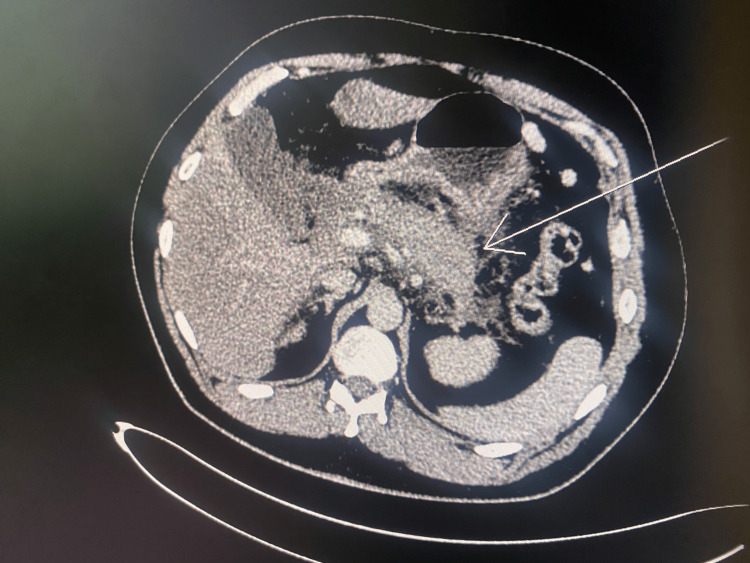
Abdominal CT scan (axial view, portal venous phase) demonstrating diffuse enlargement of the pancreas with a characteristic sausage-shaped appearance, peripancreatic fat stranding, and fluid collections consistent with Balthazar grade E acute pancreatitis. The white arrow indicates a peripancreatic fluid collection adjacent to the pancreatic tail, with surrounding inflammatory fat stranding, consistent with the extension of necroinflammation beyond the pancreatic parenchyma.
